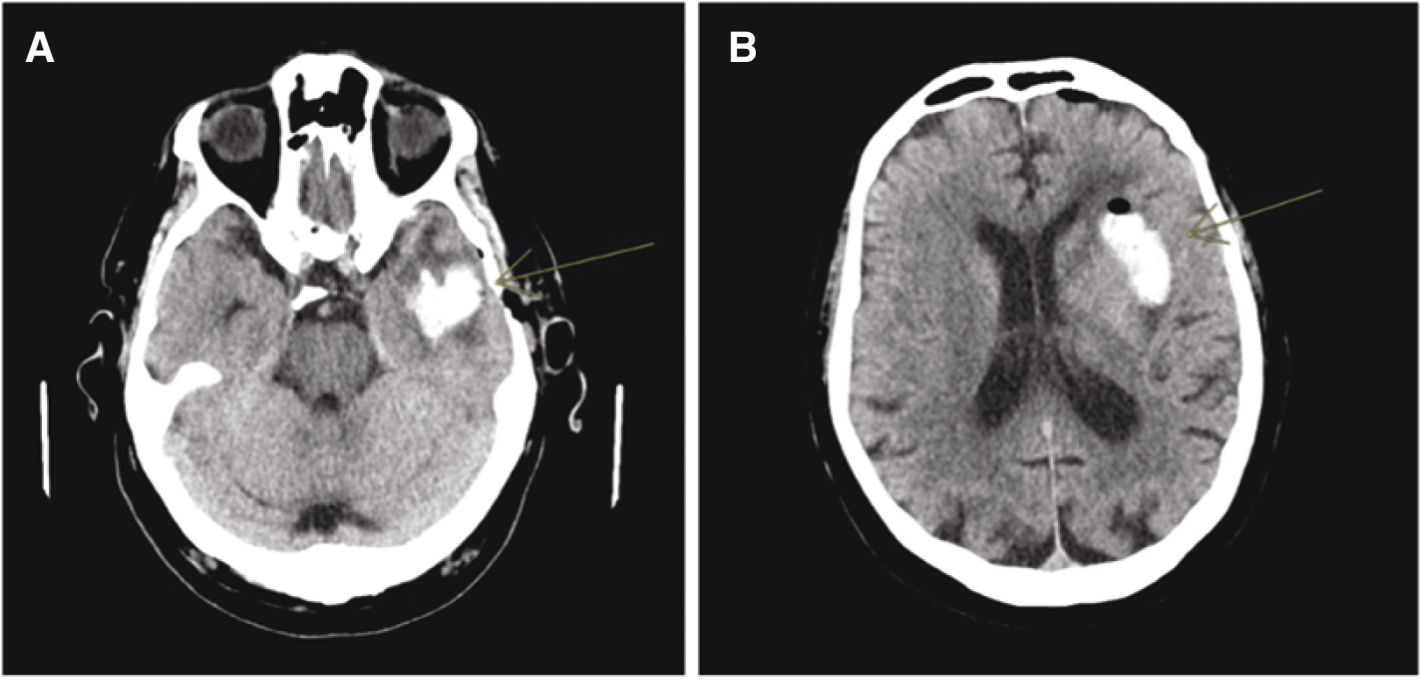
Examples of 2 postoperative hematomas on CT scan without contrast enhancement. 2A: Maximal diameter of 25 mm. 2B: Maximal diameter of 28 mm.
